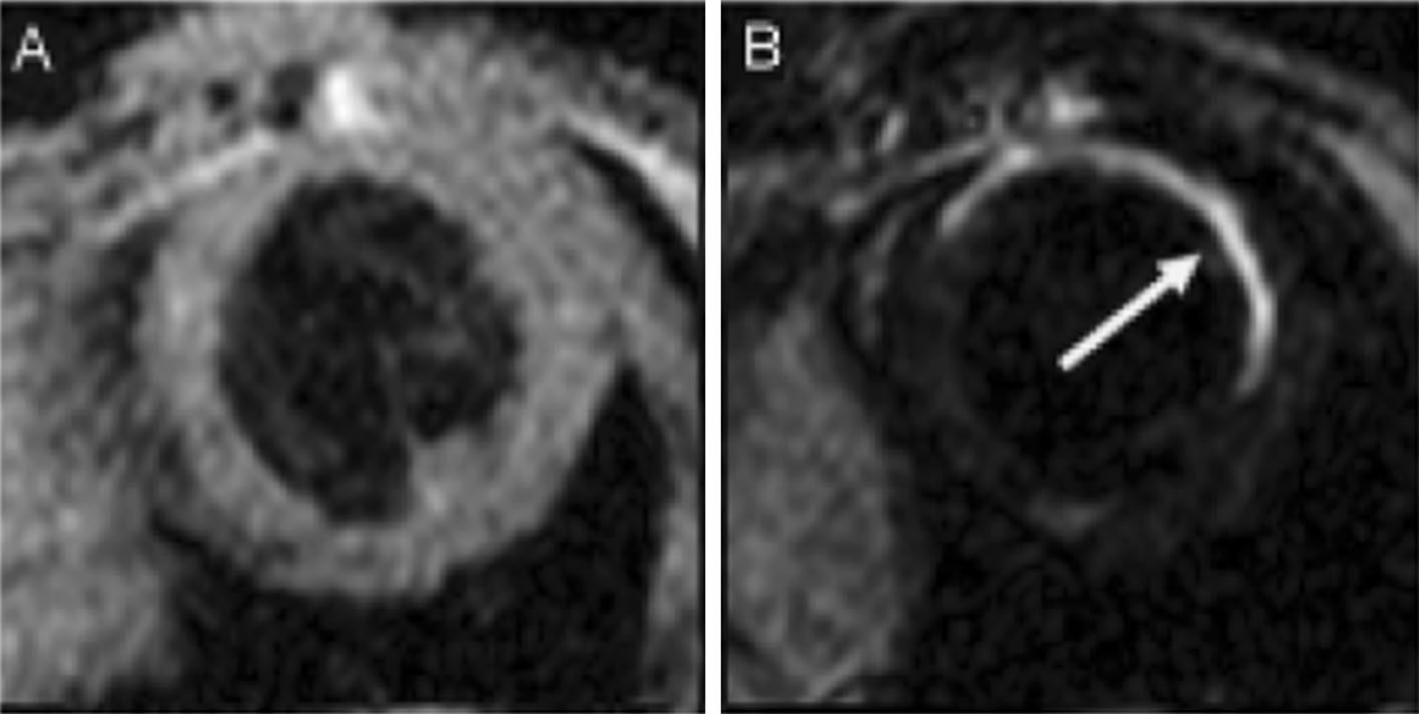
T2-weighted CMR image before injection of EP-3533 (a) and b an inversion recovery CMR image 40 min after the injection of EP-3533. The arrow highlights the hyper-intense region indicating high levels of collagen, therefore, scar [11]
